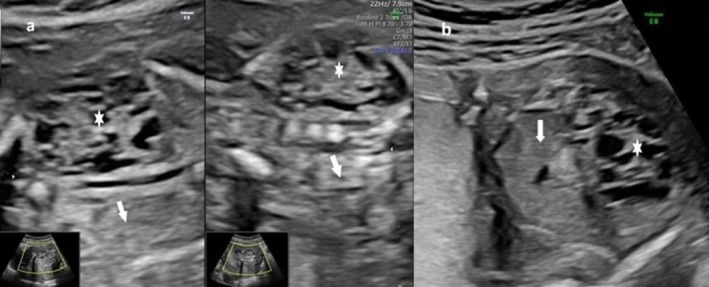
Coronal (a) and transverse (b) ultrasound view of a fetus at 20 weeks of gestational age showing the absence of the right kidney in its *loggia* (arrows) and the concomitant presence of cystic dysplasia in the left kidney (asterisk symbol).
